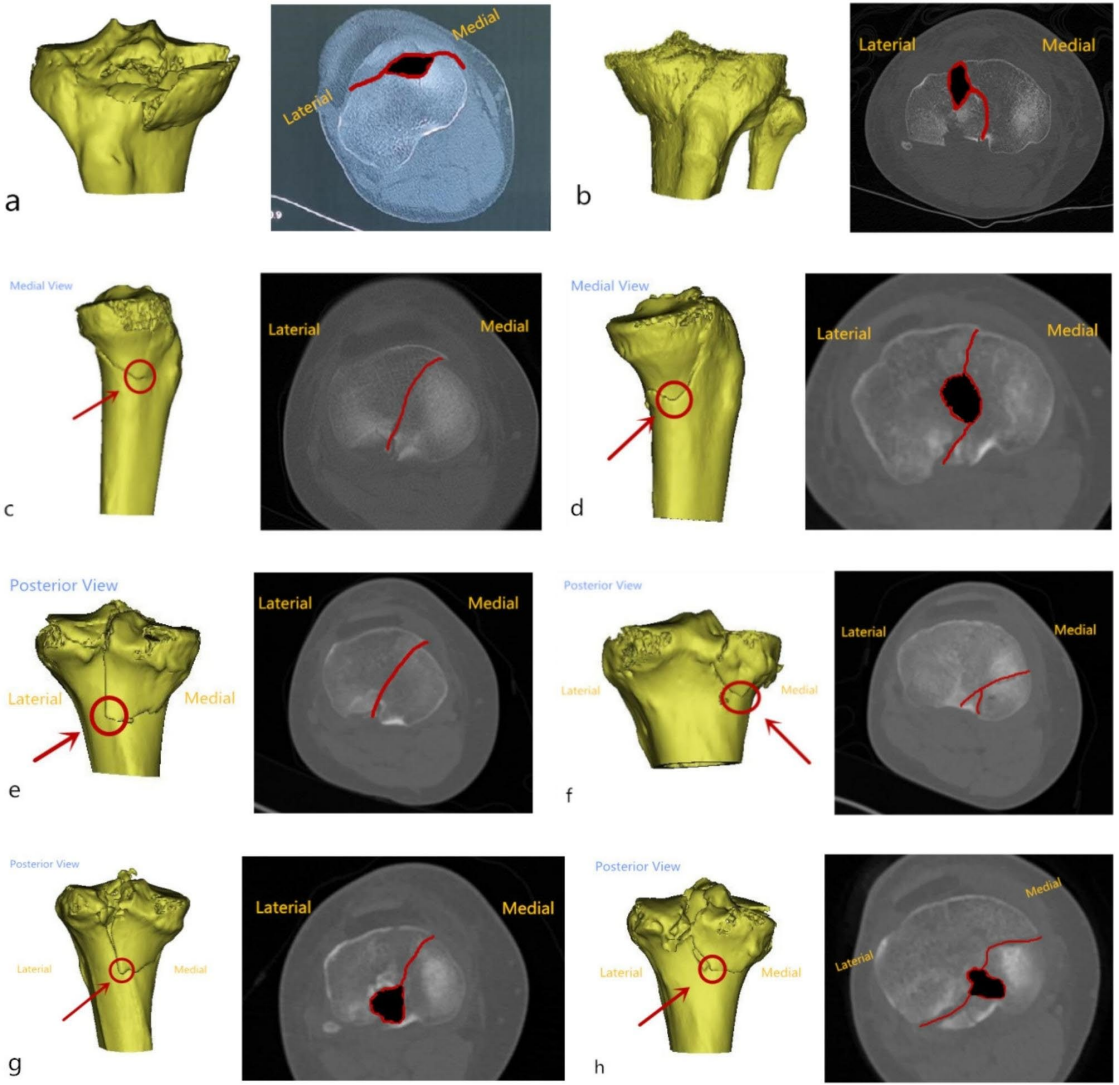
The main morphology of these 8 types was shown in CT images; the red arrows point to the MFA in 3D CT Reconstruction images, the red lines represent MOFL, and the black zones represent MCZ. (a) hyperextension-varus-internal rotation. (b) hyperextension-valgus-external rotation. (c) extension-varus. (d) extension-valgus. (e) flexion-varus-internal rotation. (f) flexion-varus-external rotation. (g) flexion-valgus-internal rotation. (h) flexion-valgus- external rotation
